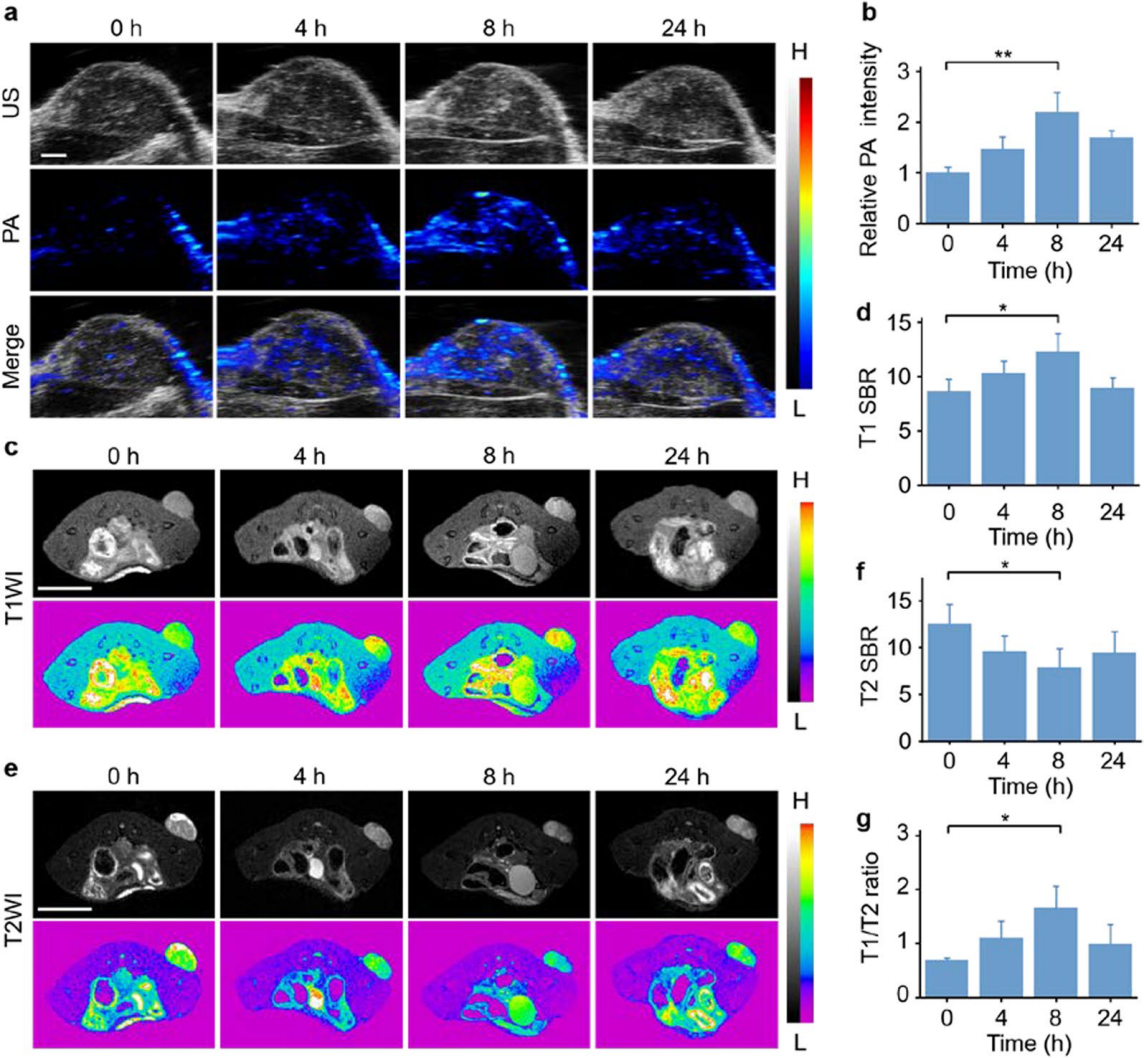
In vivo PAI and MRI of HepG2 tumors. a, b In vivo PAI of HepG2 tumors at various timepoints post-injection of FePt nanoprobes (scale bar: 1 mm) (a), and the corresponding relative photoacoustic intensities in the tumor area (b). (c, d) In vivo T1WI of HepG2 tumors at various timepoints after intravenous injection of FePt nanoprobes (scale bar: 1 cm) (c), and the corresponding T1 SBRs of the tumor area (d). e, f In vivo T2WI of HepG2 tumors at various timepoints after intravenous injection of FePt nanoprobes (scale bar: 1 cm) (e), and the corresponding T2 SBRs of the tumor areas (f). g) Ratios of T1 SBR to T2 SBR. (P* < 0.05, *P* < 0.01)
